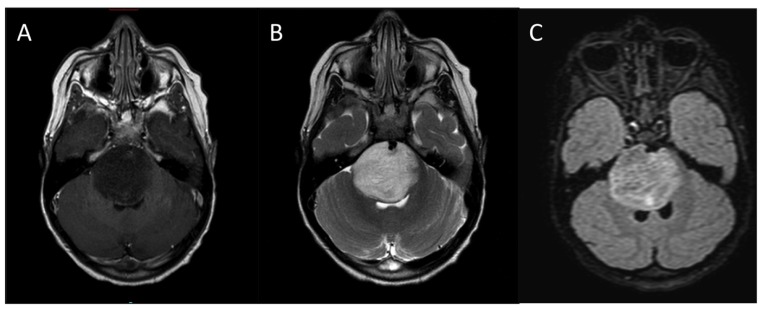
Typical MRI appearance of DIPG. (A) T1-weighted post contrast, (B) T2-weighted, (C) FLAIR.
